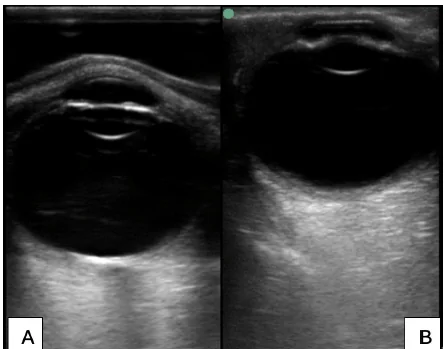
Ocular ultrasound performed using (A) modified water bath* technique and (B) conventional method. *Saline solution was used as the medium.
